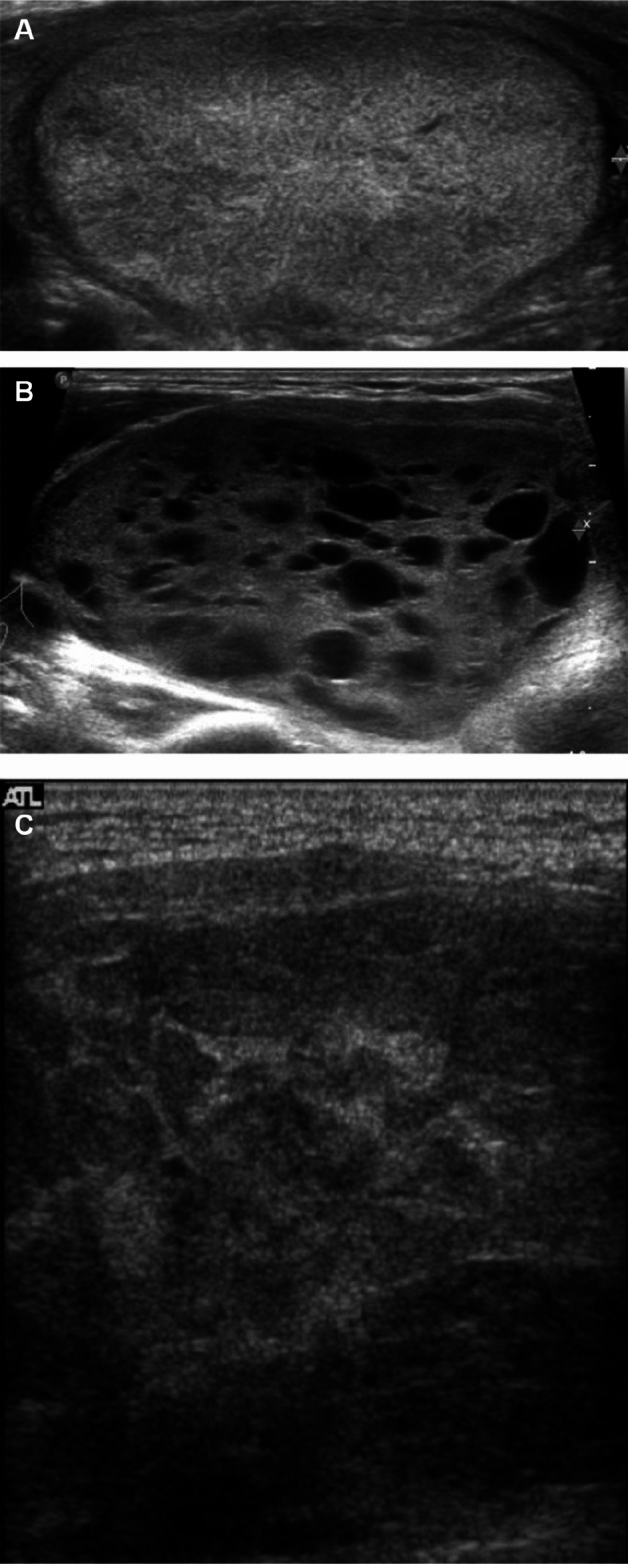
The TERT promoter wild type follicular thyroid carcinomas. Transverse ultrasound images showing a solid isoechoic nodule (A), a predominantly solid hypoechoic nodule (B), and a solid hypoechoic nodule (C) with smooth margins and no calcifications. These were classified as K-TIRADS category 3/ACR-TIRADS category 2 (A), K-TIRADS/ACR-TIRADS category 3 (B), and K-TIRADS/ACR-TIRADS category 4 (C).
